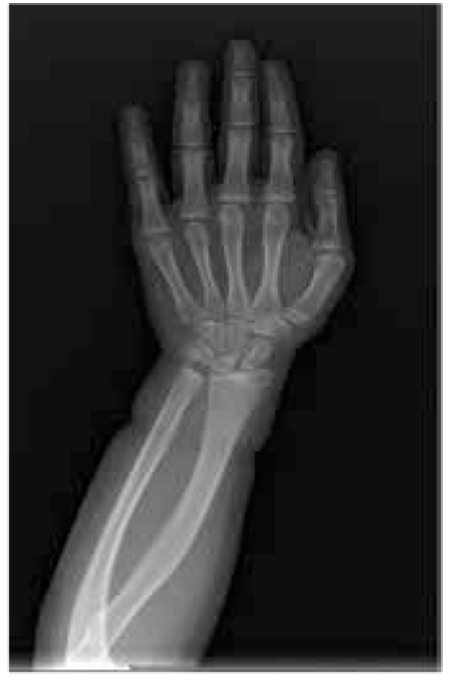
Hand and forearm radiography of the patient with SHOX deletion (P12) showing minimal bowing and mild wedging of the radius
